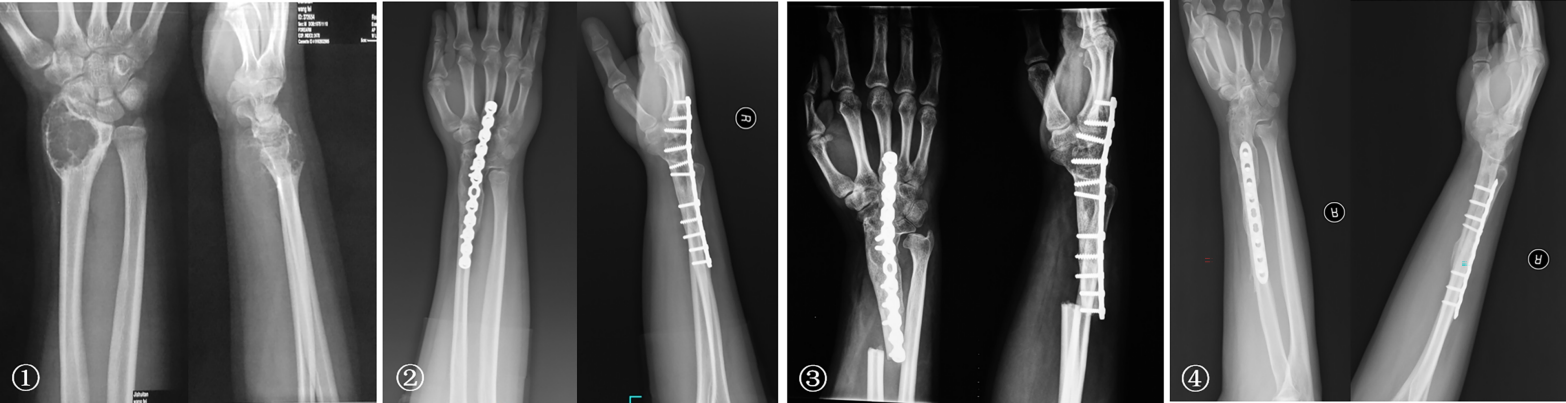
① The preoperative radiographs of a 29-year-old man with chondrosarcoma. ② Treatment included a distal radius resection and autogenous iliac bone graft with wrist joint fusion. ③ Fracture was caused by trauma eight years after surgery, and internal fixation was performed again. ④ Rotation function of the forearm is shown 192 months postoperatively.
